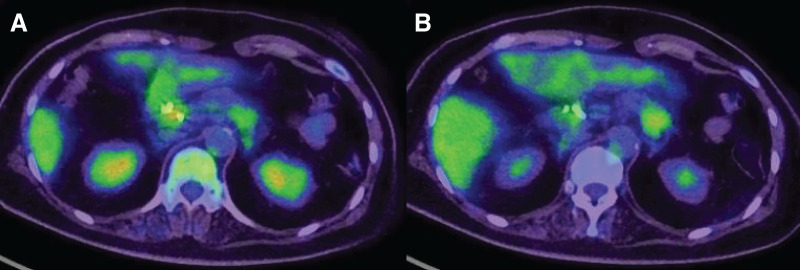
(A) PET. (B) CT. A mass lesion in the pancreatic head exhibited an SUV max of 3.8, while a mass lesion in the pancreatic tail showed an SUV max of 3.6.
